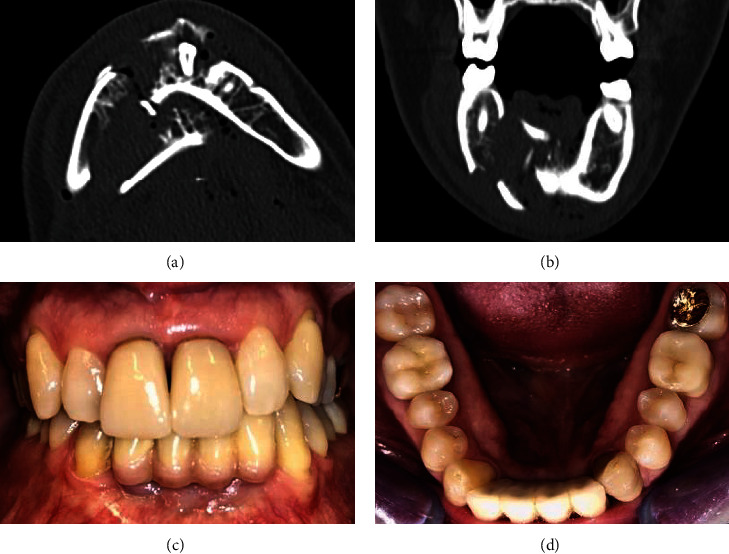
(a) CBCT horizontal view: facial skull with comminuted fracture in the mandible. (b) CBCT vertical view: facial skull with comminuted fracture in the mandible. (c) Buccal view of the clinical situation 12 years after comminuted fracture. (d) Lingual view of the clinical situation 12 years after comminuted fracture.
